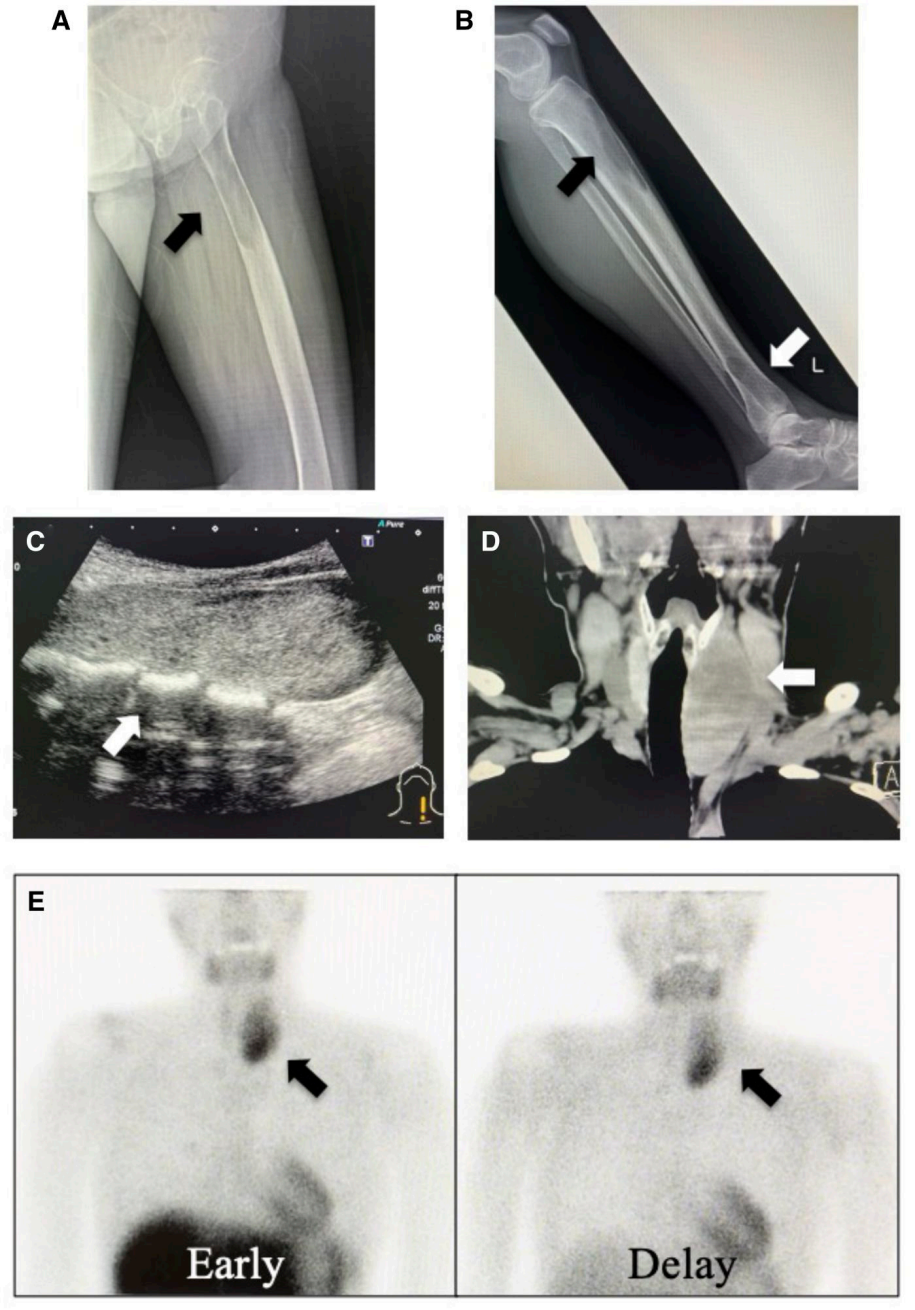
Plain radiographs of the femur (A) and tibia (B) show multiple osteolytic lesions. Neck ultrasonography (C) and computed tomography (D) show an 80 mm tumour behind the left lobe of the thyroid gland. 99mTc-MIBI scintigraphy (E) shows abnormal accumulation in the superior mediastinum. There is no accumulation in the bone lesions. 99mTc-MIBI = 99m Tc-methoxy isobutyl isonitrile.
